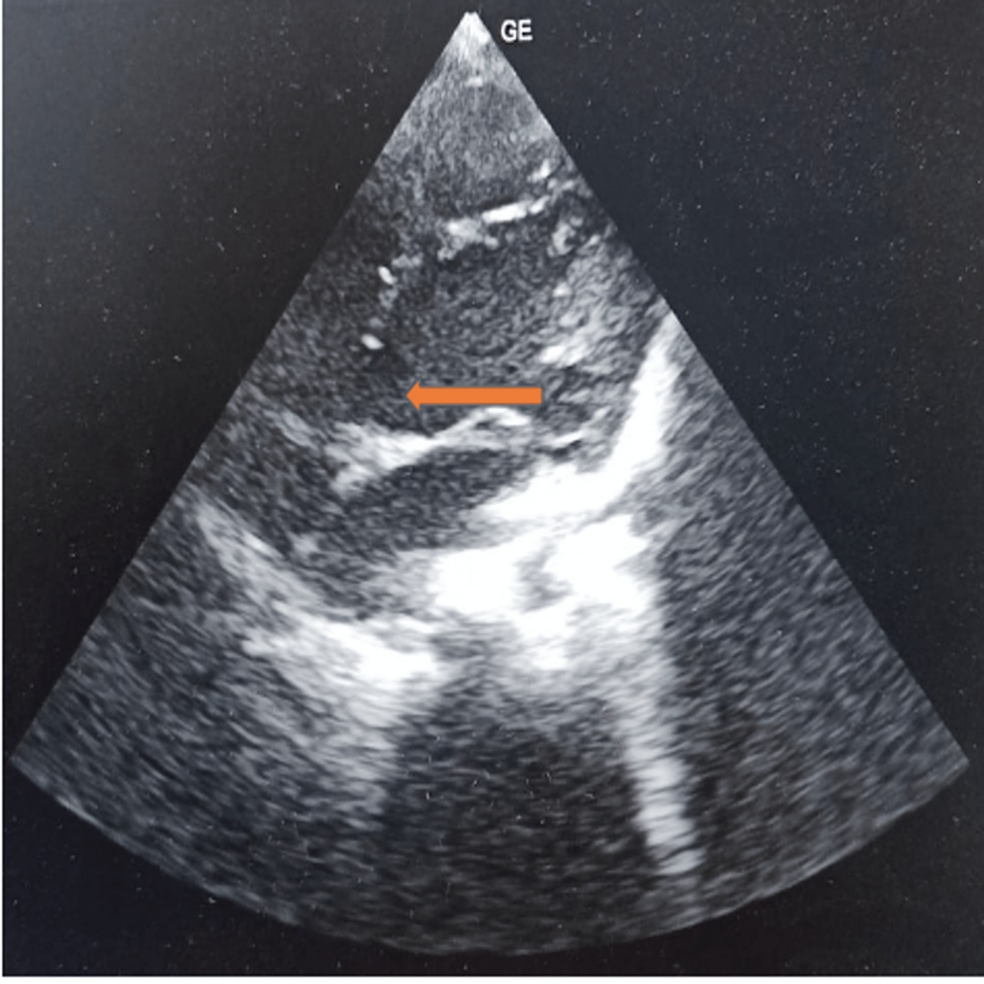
Echocardiography of the patient showing a 6 mm inlet type of ventricular septal defect on an apical four-chamber view Captured by JV
